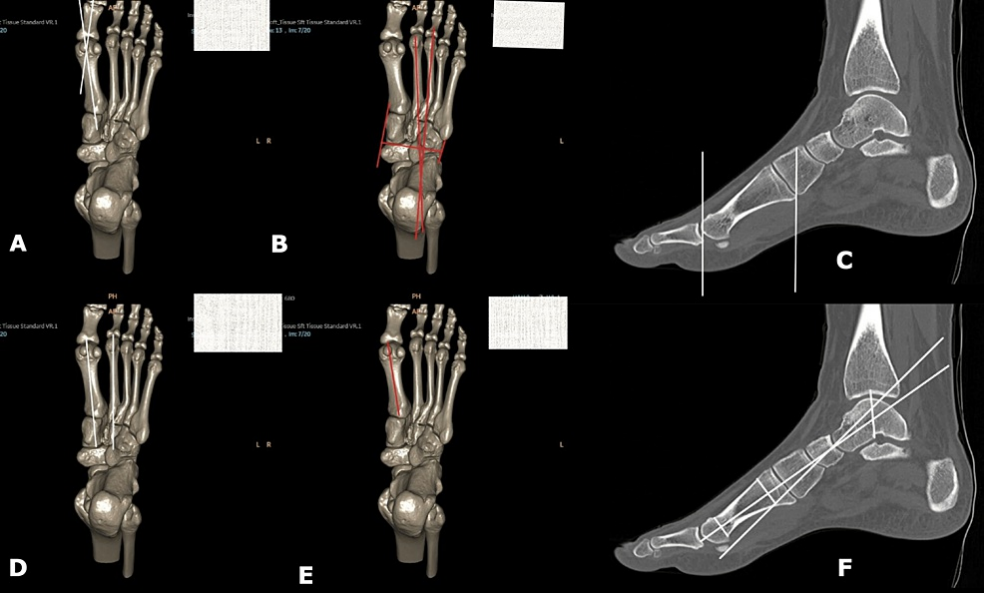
Three-dimensional reconstructed CT images (panes A, B, D, and E) and sagittal reformatted CT images (panes C and F) showing measurements for (a) hallux-valgus angle, (b) metatarsus adductus angle, (c) effective 1st metatarsal length, (d) intermetatarsal angle for hallux valgus, (e) absolute 1st metatarsal angle, and (f) Meary's angle
